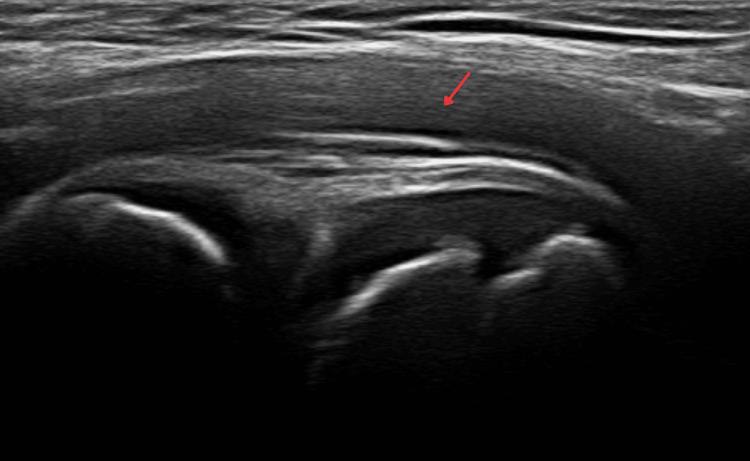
Ultrasound of the right hip joint at the time of juvenile idiopathic arthritis diagnosis. Bilateral hip joint effusion, more prominent on the right side. No Doppler power signal was detected (exudative synovitis). The remainder of the study was within normal parameters.
